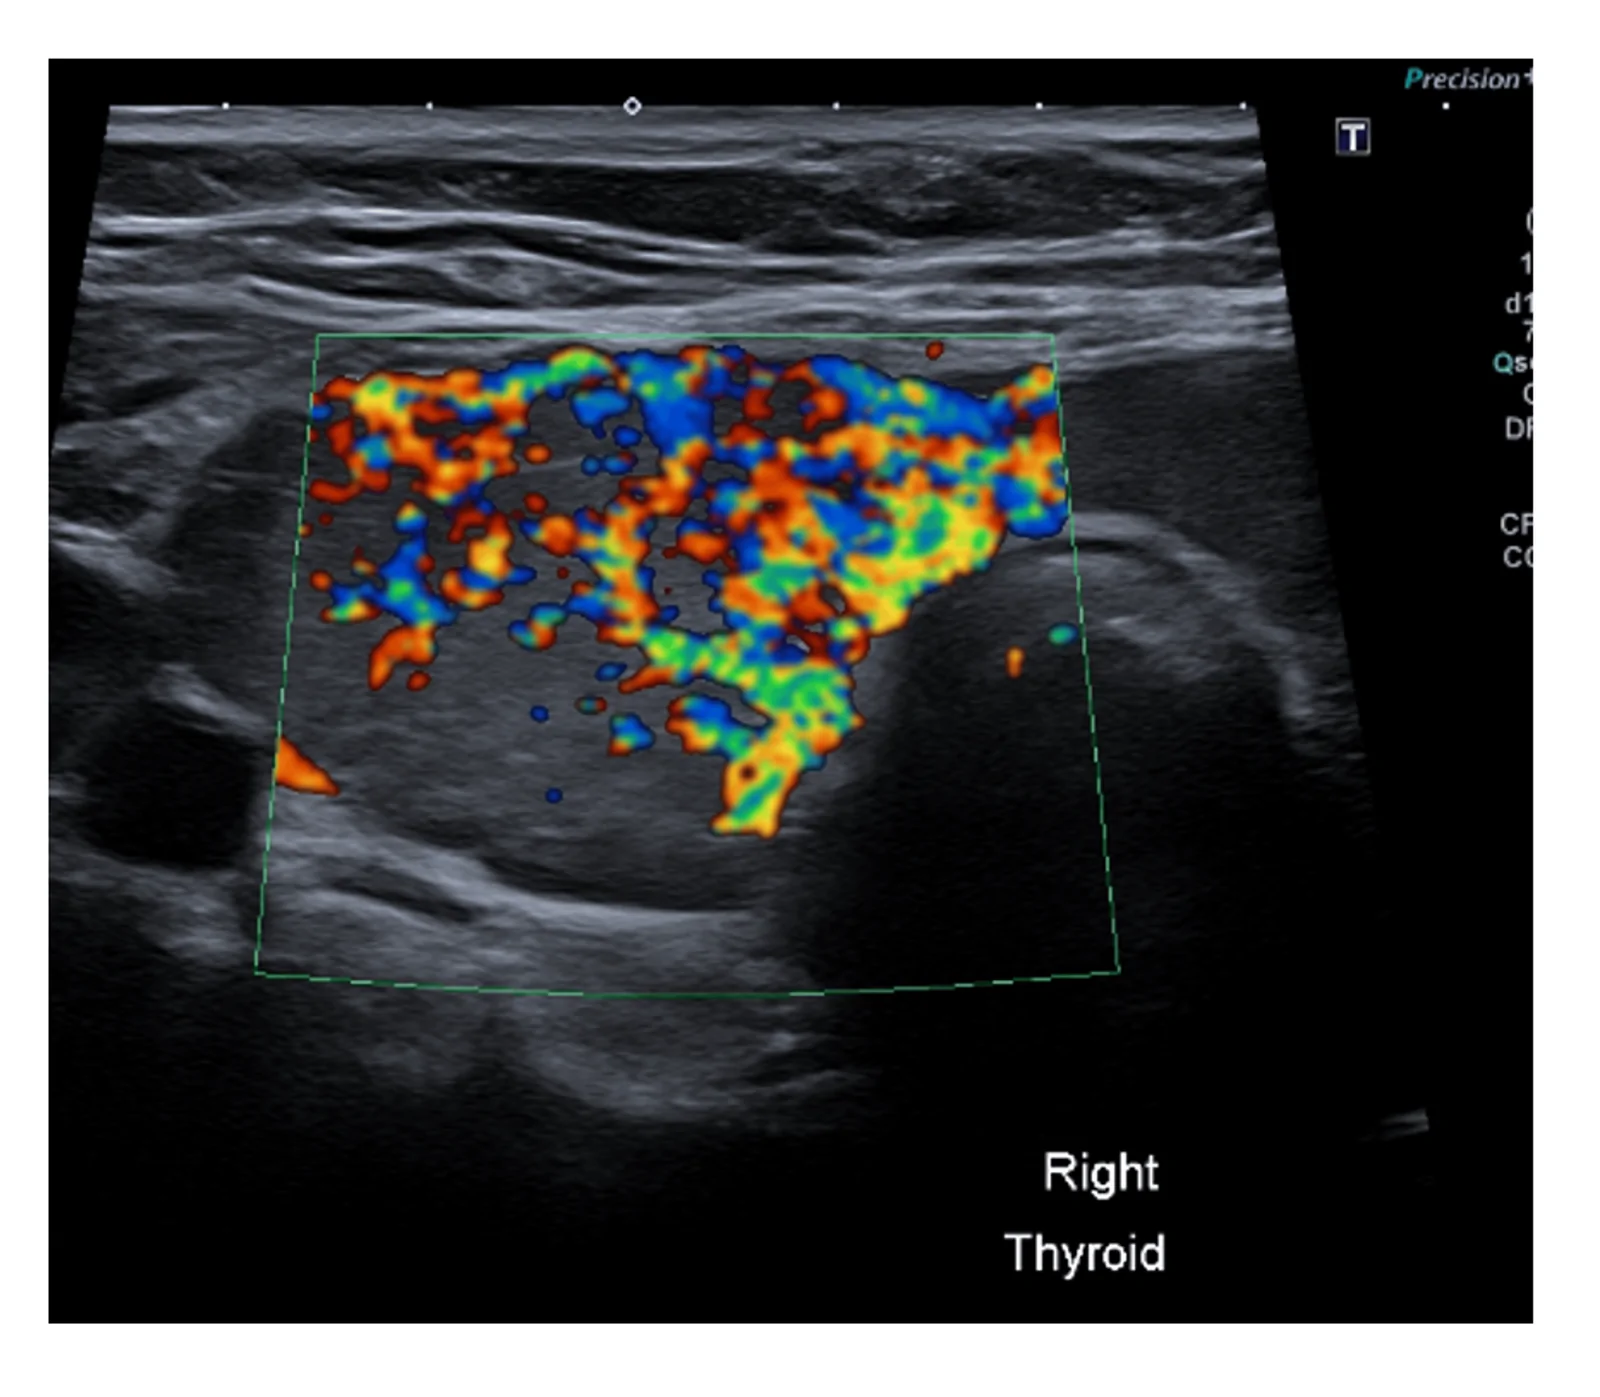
Colour Doppler Ultrasonography of the Right Thyroid Lobe Colour Doppler ultrasonography of the right thyroid lobe demonstrating diffuse thyroid enlargement with marked parenchymal hypervascularity, consistent with thyroiditis.
